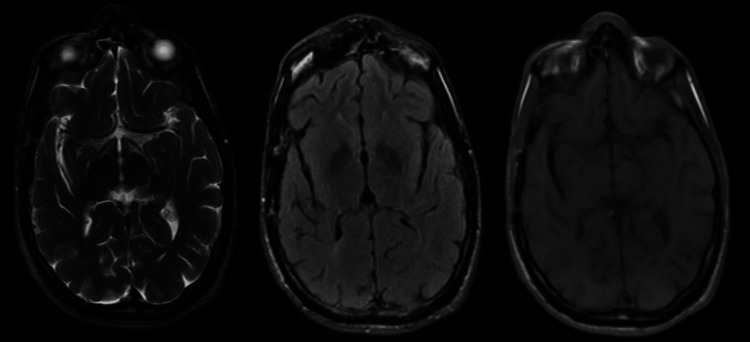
Brain MRI after 13 weeks of intravenous treatment and surgical drainage of the abscess. MRI: magnetic resonance imaging
